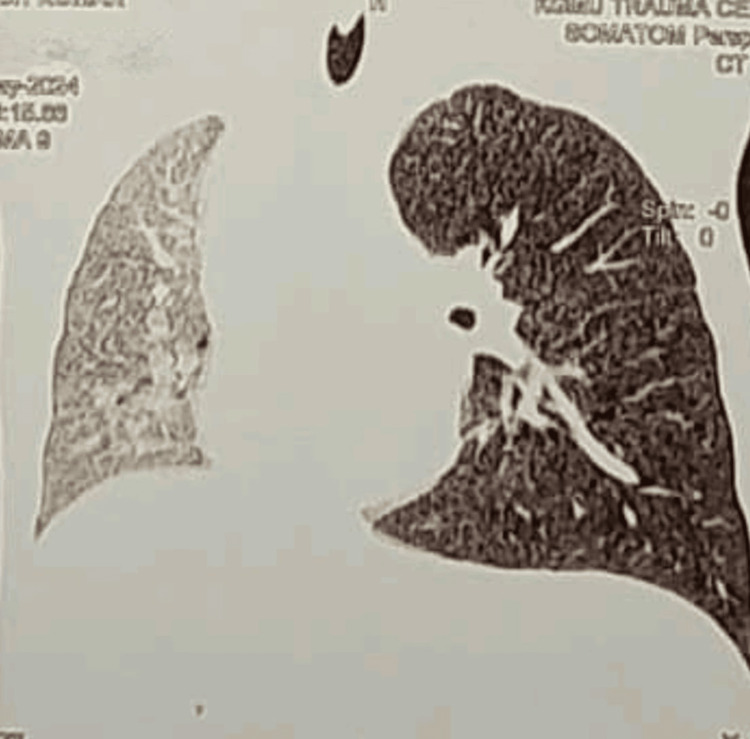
Contrast-enhanced CT thorax, coronal view Window for pulmonary parenchyma in coronal view showing ground glass attenuation diffusely in the right lung with overinflation of the left lung.
